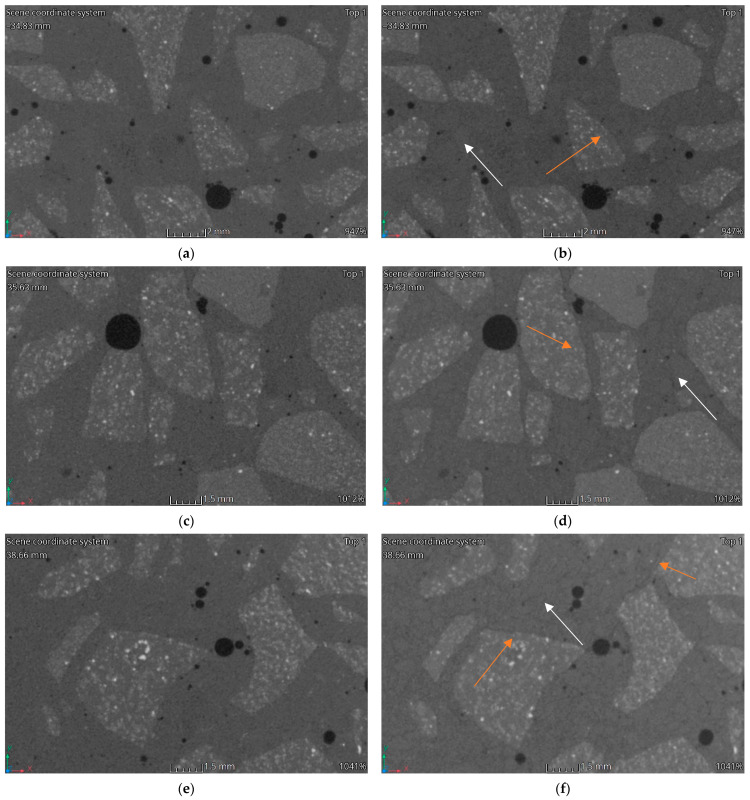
CT imaging results. Structural changes in three samples HPFRC 0.9: (a) 20 °C; (b) 200 °C; (c) 20 °C; (d) 400 °C; (e) 20 °C; (f) 600 °C.
